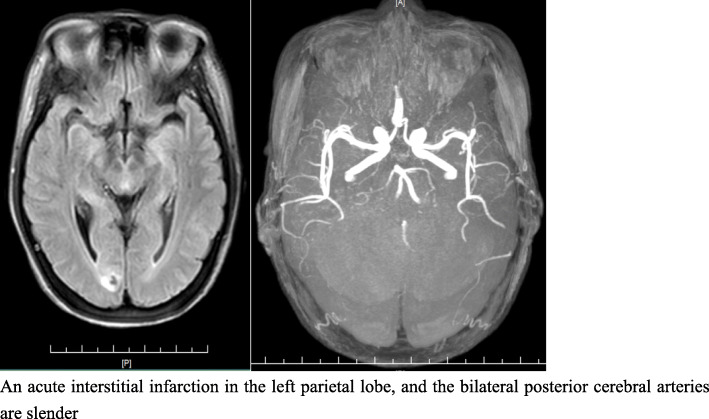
MRI + MRA images: An acute interstitial infarction in the left parietal lobe, and the bilateral posterior cerebral arteries are slender
